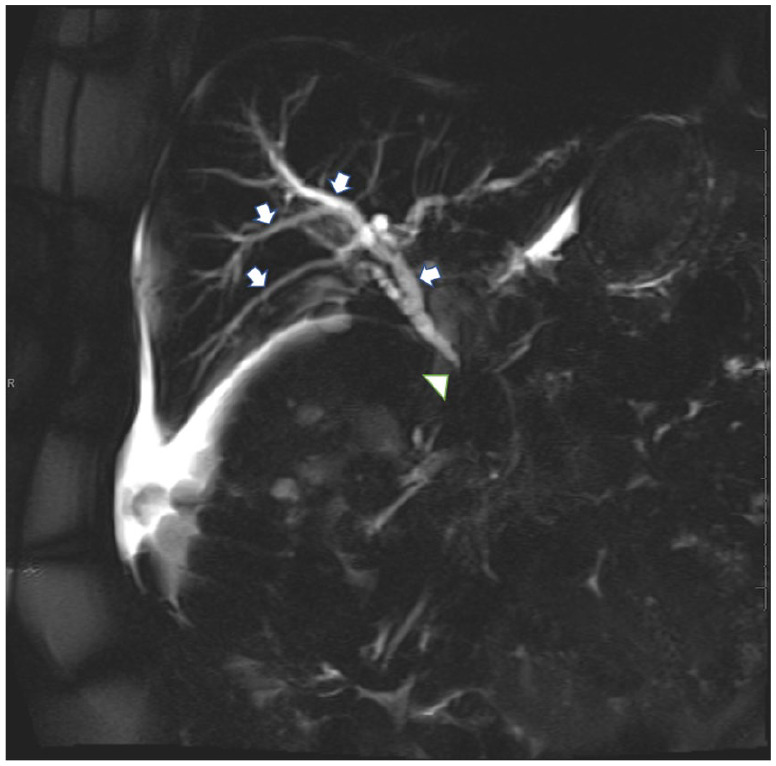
Single shot fast spin echo magnetic resonance image shows intrahepatic and extrahepatic bile duct dilatation (white arrows) with abrupt narrowing at the head of the pancreas (white arrowhead), compatible with biliary obstruction.
